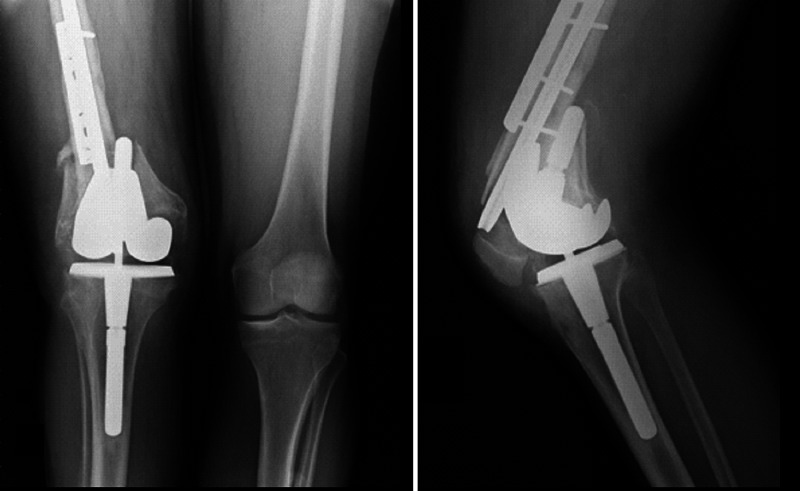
Anteroposterior and lateral knee X-rays taken two years after the second distal femoral allograft implantation. A supracondylar-intercondylar fracture is observed in association with the structural allograft failure.
